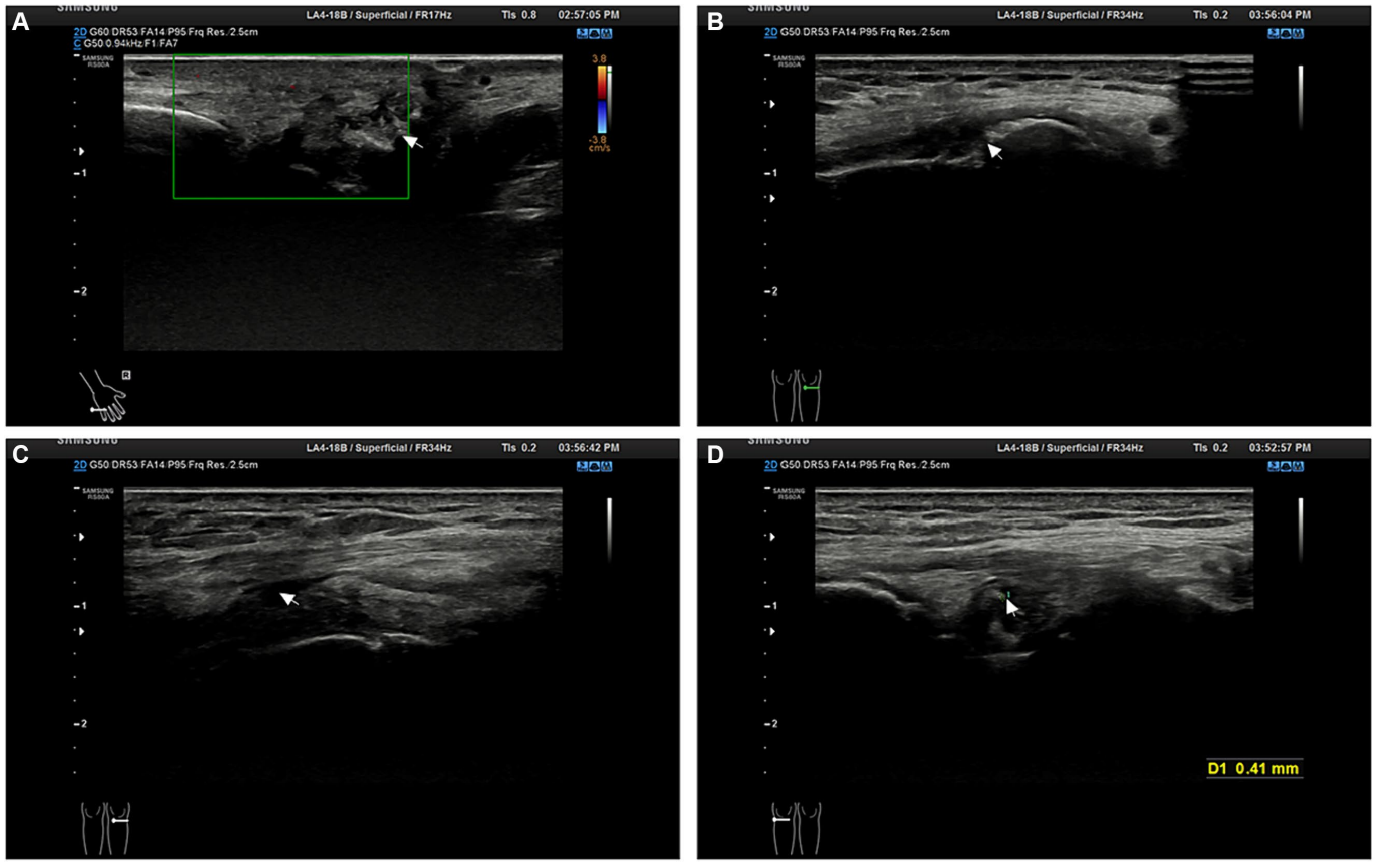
Patient joint ultrasonography images. (A) The image shows synovial proliferation with bone erosion in the interphalangeal joints of the right hand. (B) The image suggests bone erosion in the knee joint. (C) The image reveals knee joint effusion. (D) The image demonstrates the formation of tophi (size *D* = 0.41 mm, indicated by the arrow).
